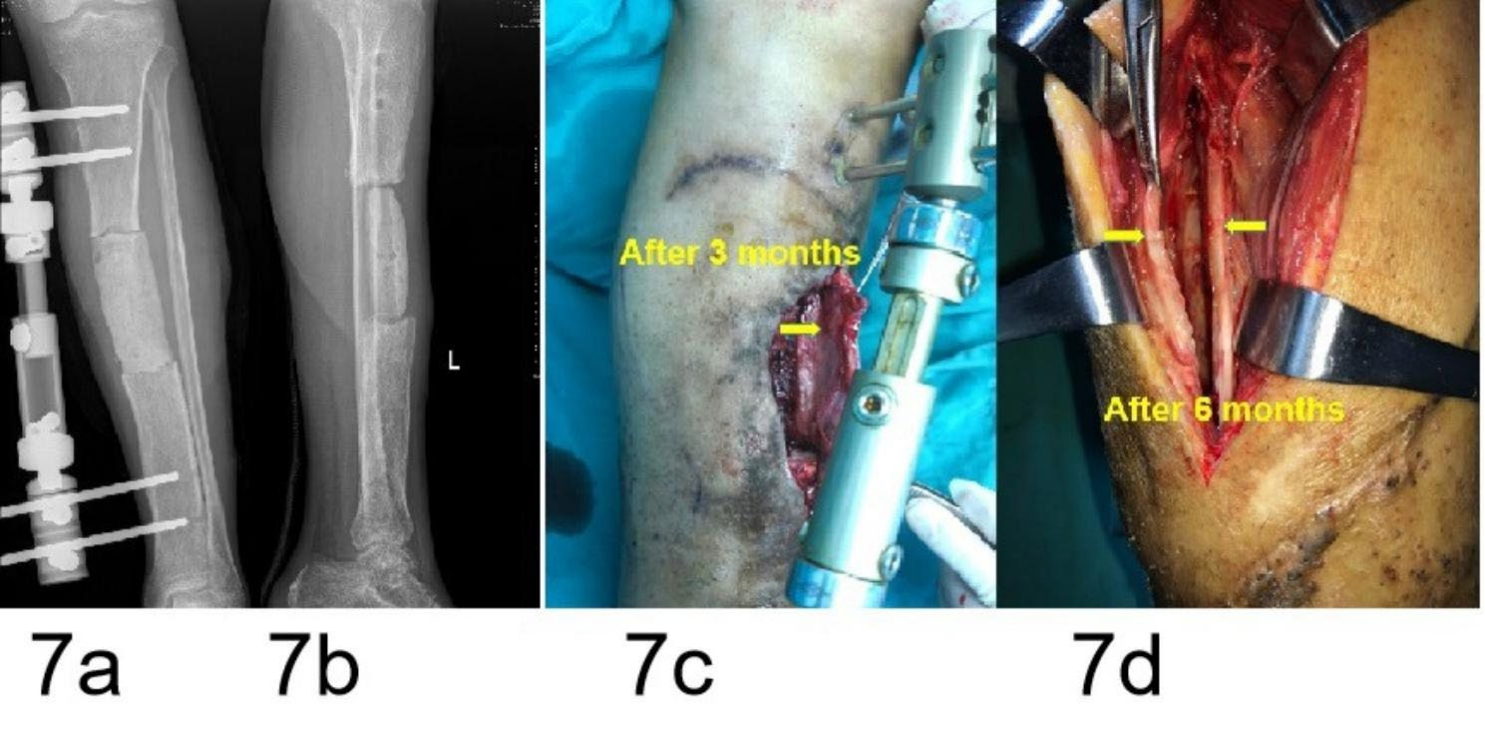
A 29-year-old male patient with a grade I IMSO. (a,b) AP and lateral radiographs showing the cement spacer of PMMA inserted in the tibial defects after resection due to osteomyelitis. (c) Intraoperative exploration revealing the IM (yellow arrow) was thin (1 mm) at three months after insertion of the cement spacer. (d) Intraoperative exploration revealing the IM was thicker (2–3 mm) and harder (yellow arrow) at six months compared to three months
